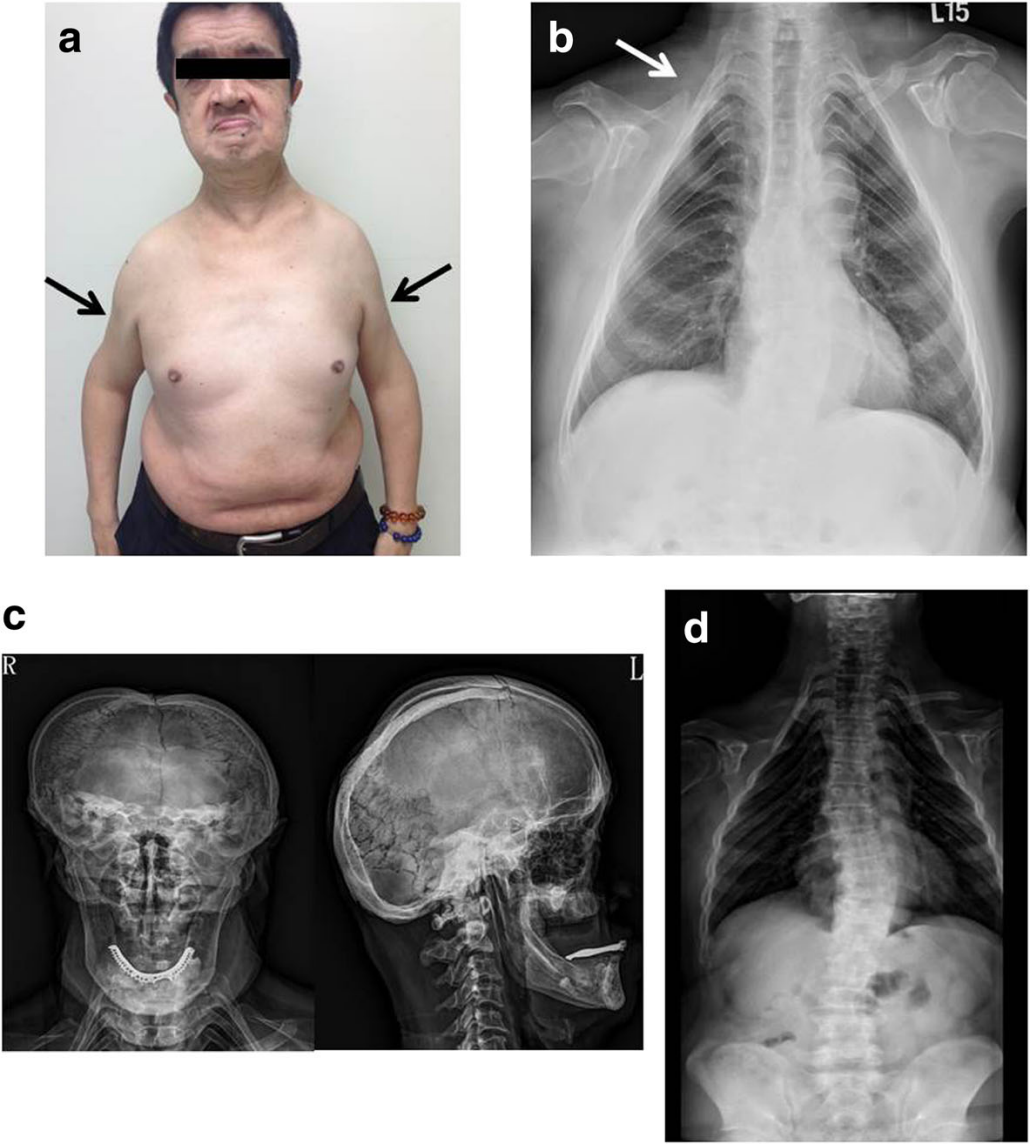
Clinical pictures and radiologic findings for the index patient with CCD. a The photographs show a flat face, sunken nasal bridge, hypertelorism, chin protrusion, and sloping shoulders. Arrows indicate atrophy of bilateral shoulder girdle muscles. b Chest X-ray shows the absent right clavicle (arrow) and a hypoplastic left clavicle. c Skull X-ray shows wormian bones in the skull (left panel) and a hypoplastic maxilla (right panel). d Spine X-ray reveals mild scoliosis of the thoraco-lumbar spine
